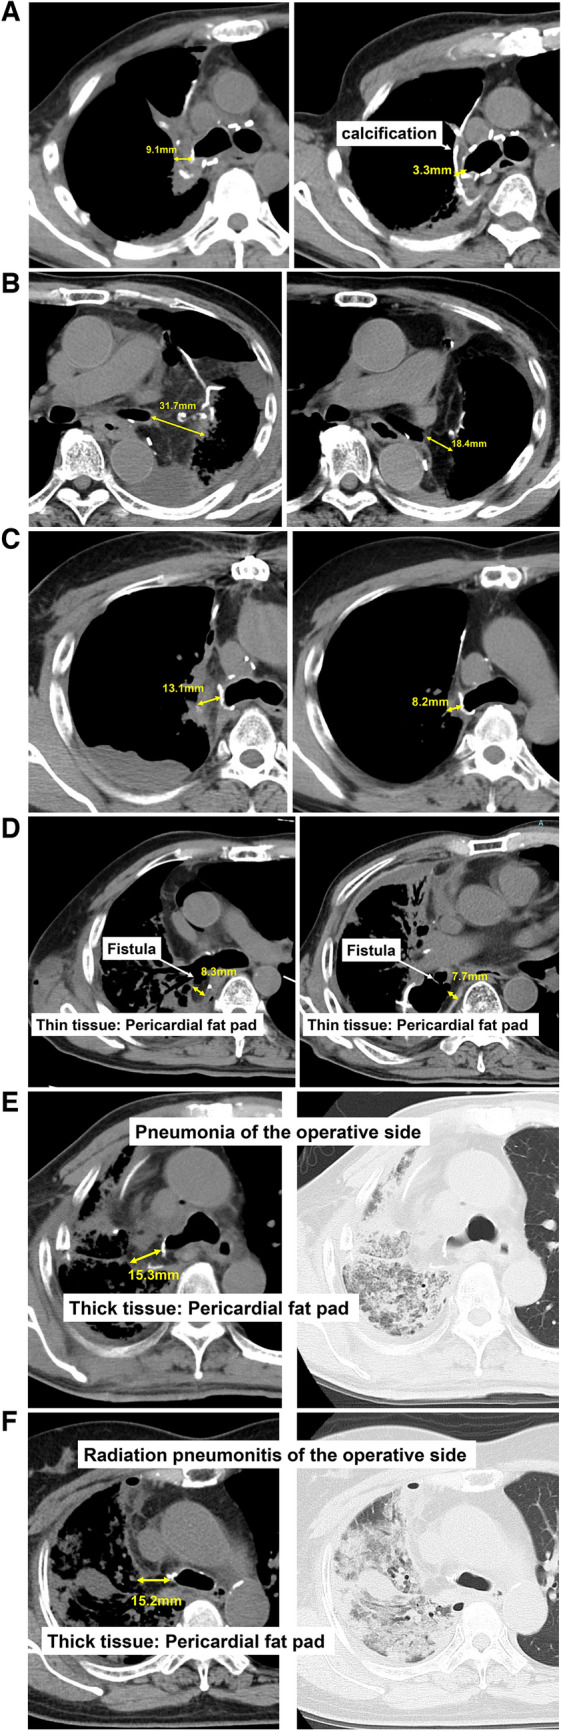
CT scans of patients in whom the bronchial stump/anastomotic site was covered by A intercostal muscle, B omentum, and C pericardial fat pad. The arrow shows the thickness of the tissue flap covering the bronchial stump/anastomotic site in the transverse plane on CT scans obtained within 1 month (left side) and approximately 1 year after surgery (right side). D In the cases in which BPF developed (left side: case No.3, right side: case No.5), the thickness of the covering tissue were 8.3 mm and 7.7 mm, respectively. E, F In the cases without BPF, irrespective of postoperative E pneumonia and F radiation pneumonitis of the operative side (considered to be risk factors for BPF), the thickness of the covering tissue was 15.3 and 15.2 mm, respectively (left side: mediastinal window setting, right side: lung window setting)
